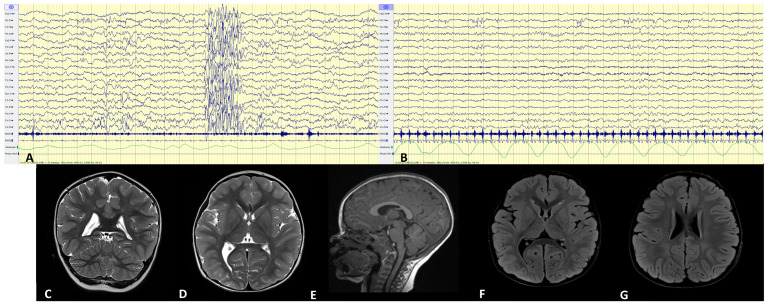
Sleep EEGs show, during drowsiness, (A) generalized discharges of slow, sharp, high-voltage waves and spike waves, with no clinical correlate; during wakefulness and sleep, (B) spike waves in the right fronto-temporal regions, which increase during drowsiness and sleep. All EEGs are shown at filter settings: high pass of 0.5 Hz, low pass of 40 Hz, notch of 50 Hz, and time base of 15 mm/sec. The sensitivity is 200 μV/cm (A,B). Imaging studies with MRI at the age of 3 years (C–G). Coronal (C) and axial (D) T2-weighted images show a normal ventricular system with moderate asymmetry of the lateral ventricles, particularly in the posterior portions, with the right side being larger than the left. Sagittal (E) T1-weighted image shows a normal cerebellum and corpus callosum. Axial (F,G) FLAIR images show slight hyperintensity affecting the deep superotrigonal white matter bilaterally, not pathological and related to the presence of dilated perivascular spaces; previously described ventricular asymmetry is also evident.
